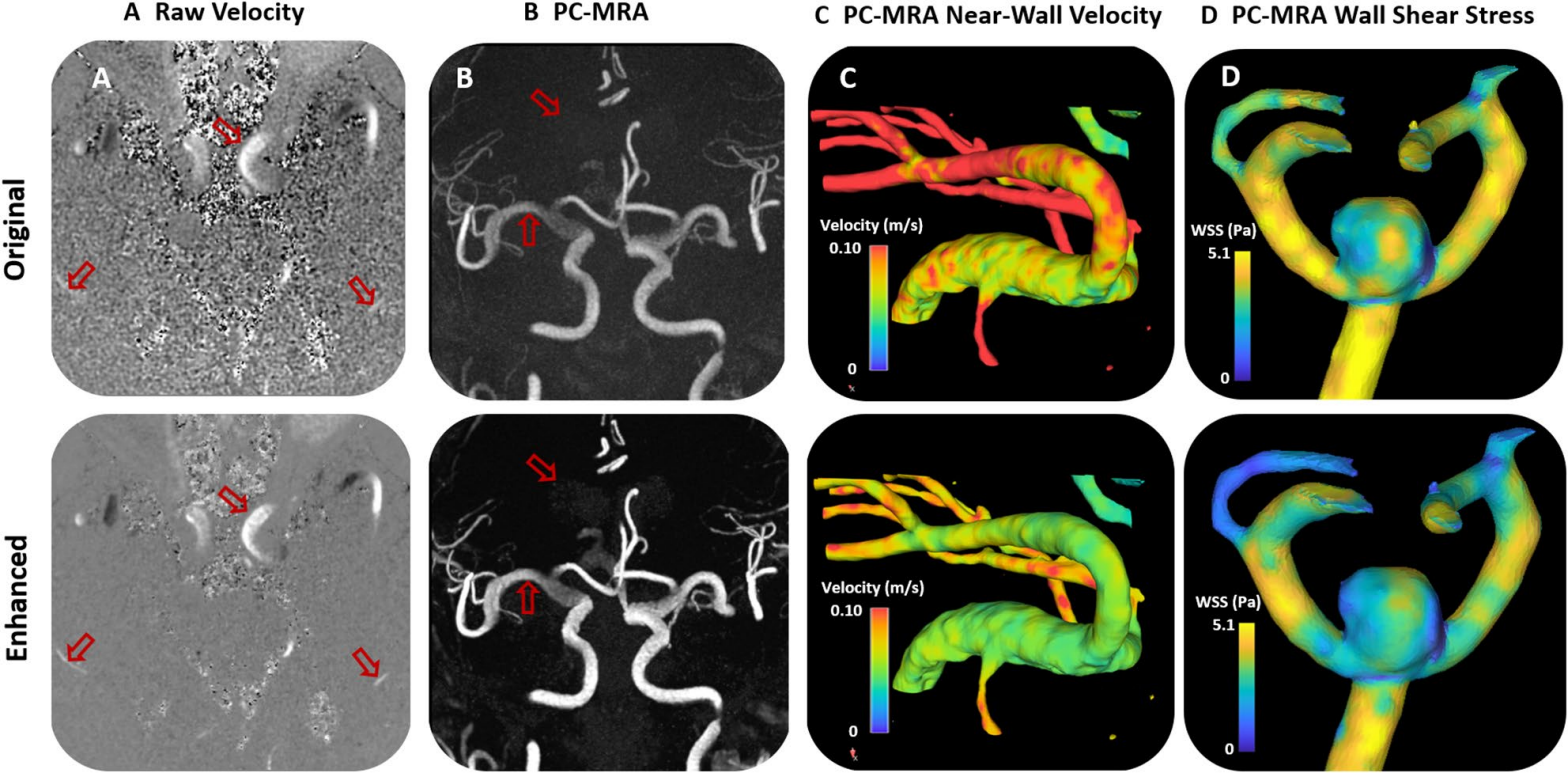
Patient cerebral flow MRI results. (a) Cerebral 4D flow MRI raw velocity images before and after trained network application. (b) Phase-contrast MR angiogram images before and after trained network application. (c) Near wall velocity contours displayed on cerebrovascular segmentations before and after network application. (d) Wall shear stress plots on a cerebral aneurysm wall. Arrows note key areas of image improvement.
